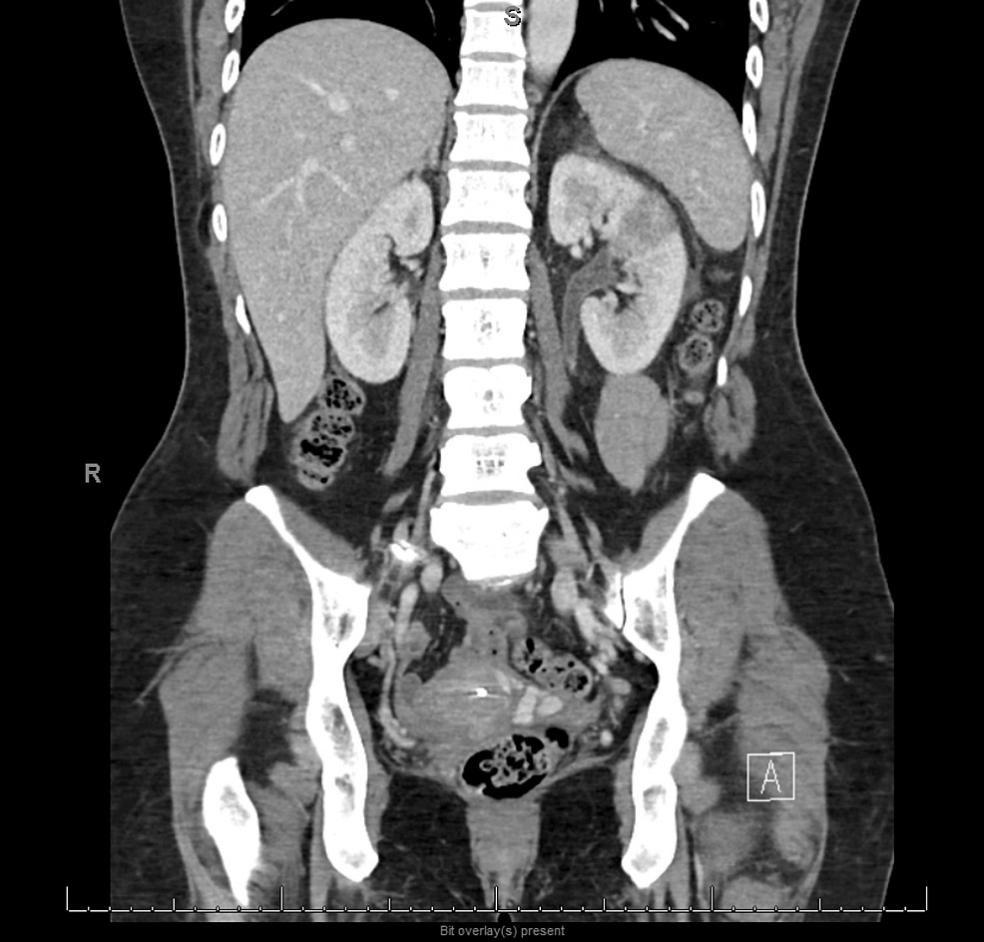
CT scan of abdomen and pelvis showing mild left ureteral wall thickening secondary to ureteritis
